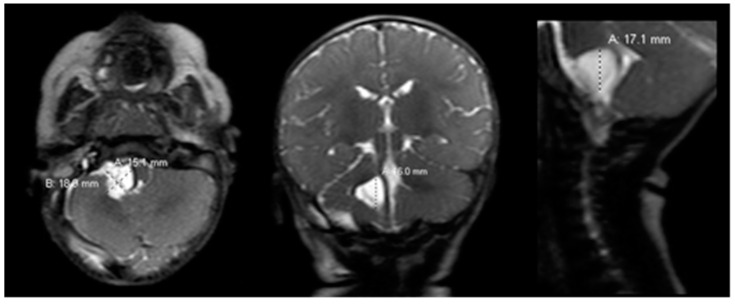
Postoperative brain MRI scan. The postoperative brain MRI scan on postoperative day 1 (left = axial; middle = coronal; right = sagittal) T2 sequences showed interval decompression of the prior known arachnoid cyst with decreased mass effect. MRI = magnetic resonance imaging.
